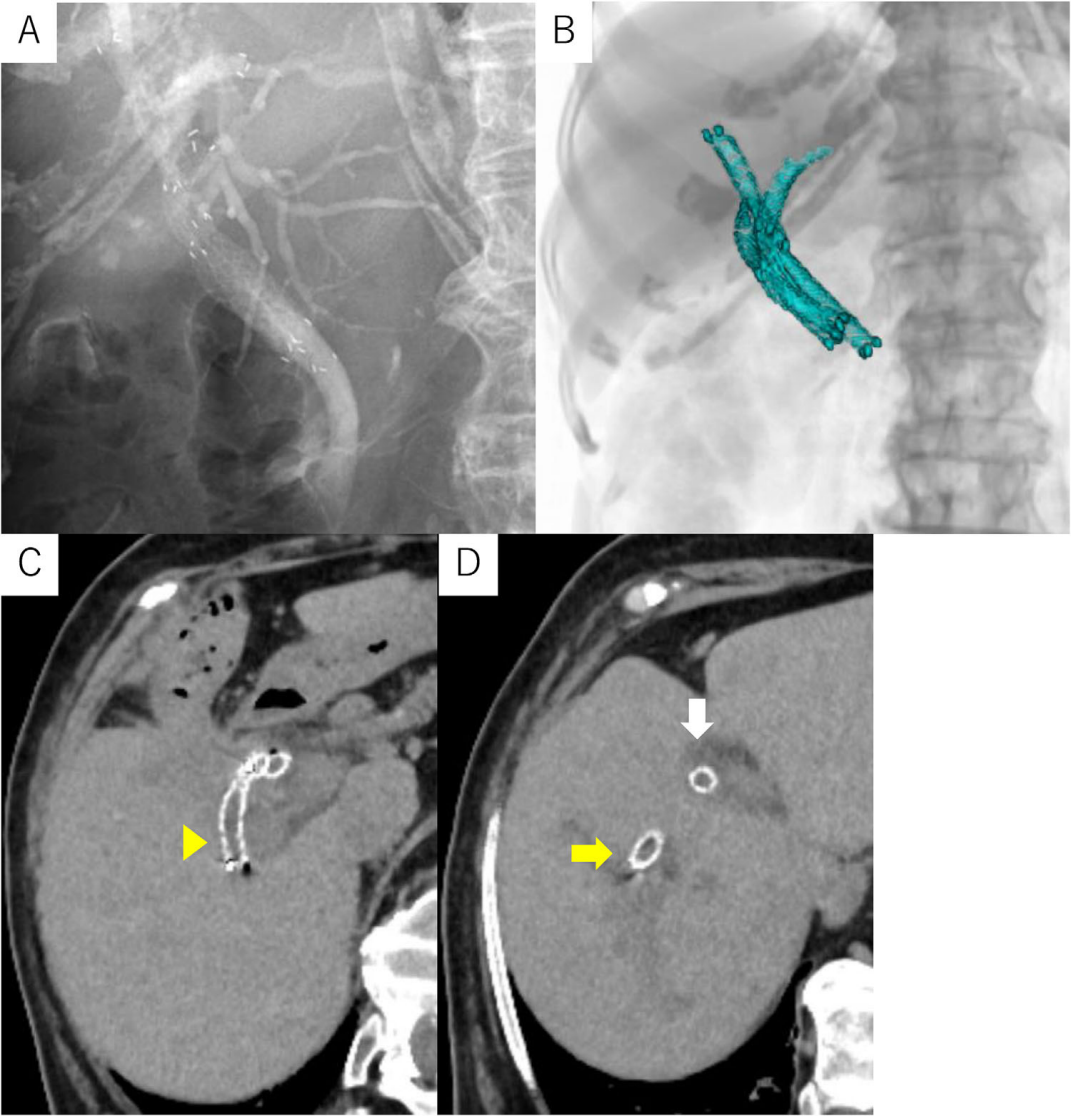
Fluoroscopic image after simultaneous deployment of three MHSEMSs. (A, B) Three‐dimensional reconstruction from post‐procedure CT demonstrating the configuration of deployed stents within the intrahepatic ducts. (C, D) Post‐procedural axial CT demonstrating correct placement of the three MHSEMSs in the right anterior (yellow arrow), right posterior (yellow arrowhead), and left hepatic ducts (white arrow). MHSEMSs: multi‐hole self‐expandable metallic stents, CT: computed tomography.
